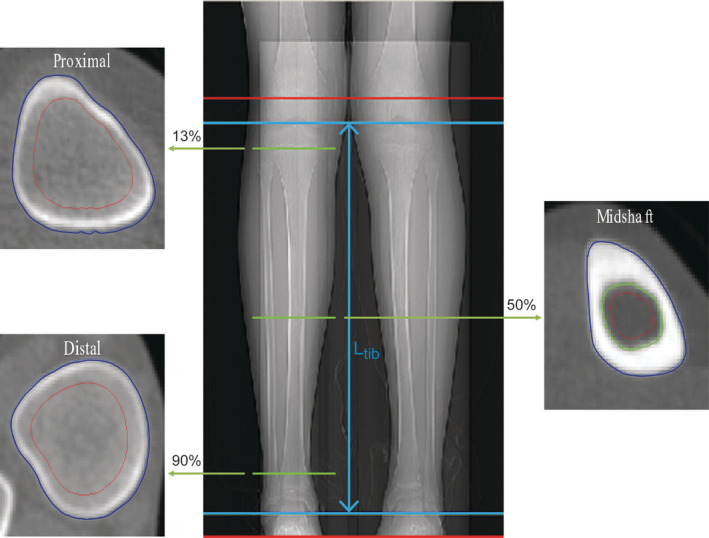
CT scan and analysis regions. Cross‐sectional area was measured as the area within the periosteum (blue contour) for all regions, and density‐weighted cross‐sectional area of the metaphyses was measured in the same region. Cortical bone area was measured as the cortical region between the periosteum and endosteum (blue and green contours), and cortical thickness, density, and moments of inertia were calculated using the same region. Cancellous density was measured within a region shrunk 30% from the blue contour and centered around the original geometric center (red contour).
